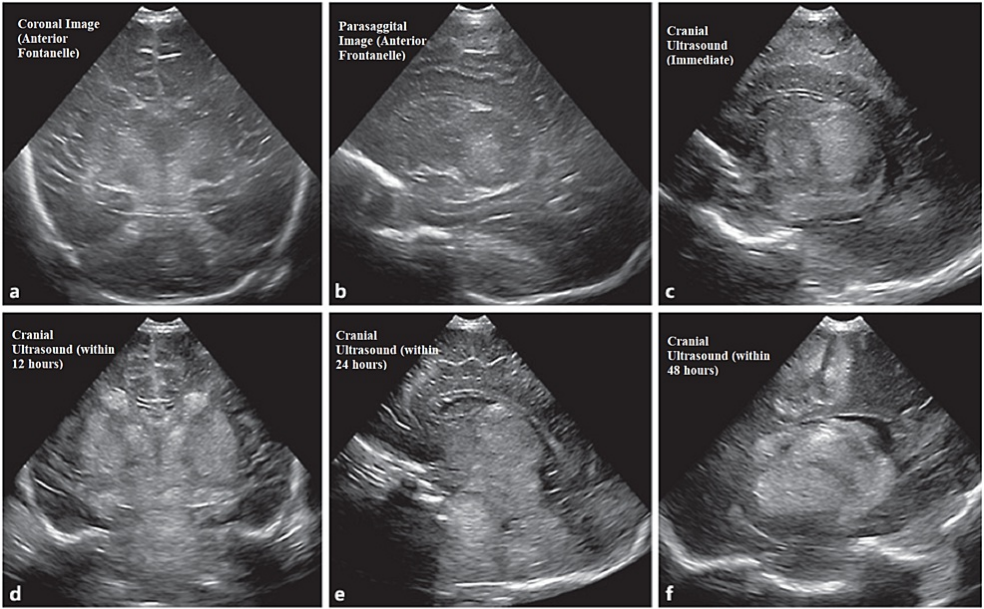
cUS with distinctive imaging pattern in HIE. (a) Full-term neonate with coronal image through AF; (b) full-term neonate with parasagittal image through AF; (c) cUS showing ischemic injury marked by bilateral symmetric increased echogenicity in thalami shows a central pattern of injury; (d) ventricles are slit-like due to edema from the central structures within (d) 12 hours of cUS, (e) 24 hours of cUS, and (f) 48 hours of cUS. AF: anterior fontanelle; cUS: cranial ultrasound; HIE: hypoxic-ischemic encephalopathy.
